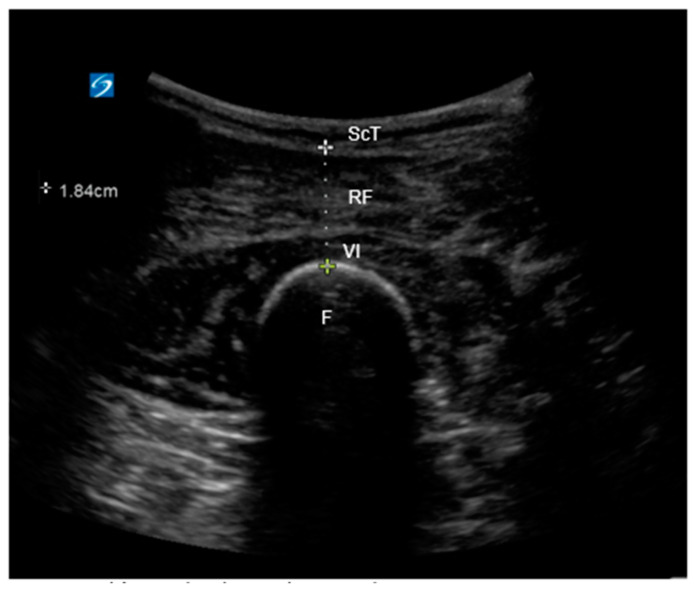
Quadriceps depth on an ultrasound image. The quadriceps depth was obtained by measuring the distance between the cortex of the femur and the most superficial muscular fascia. ScT = subcutaneous tissue; RF = rectus femoris; VI = vastus intermedius; F = femur.
